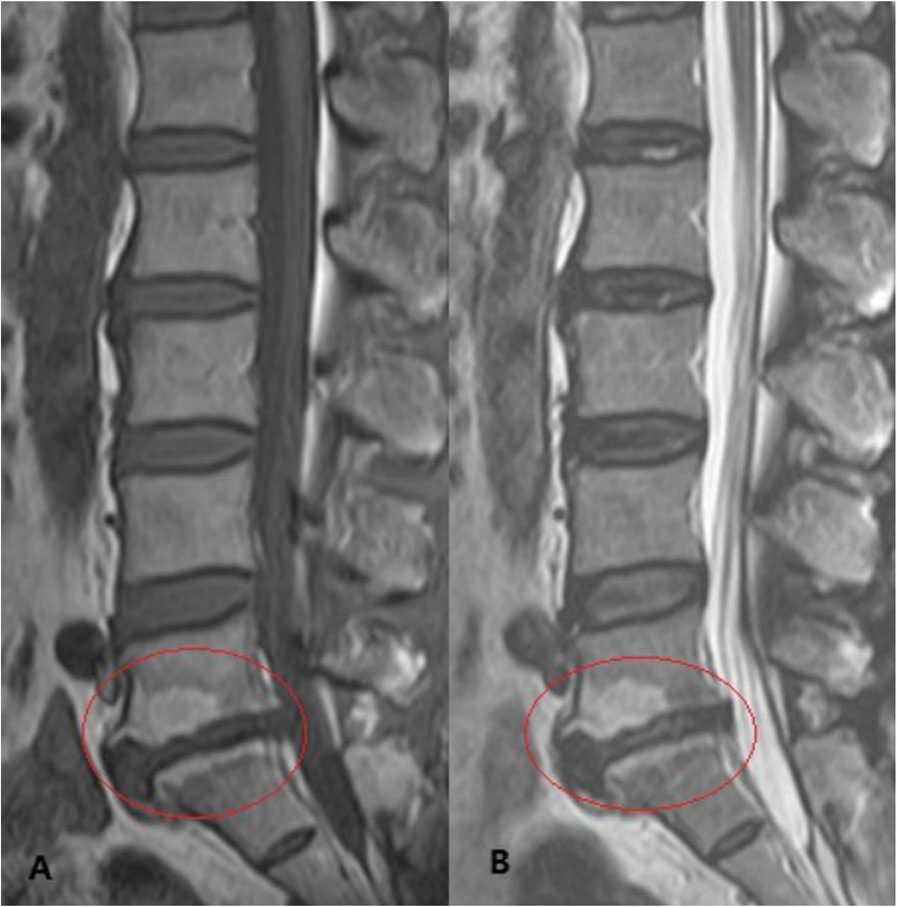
Modic type 2 change. a Hyperintensity on T1-weighted images at the L5/S1. b Hyperintensity on T2-weighted images at the L5/S1
